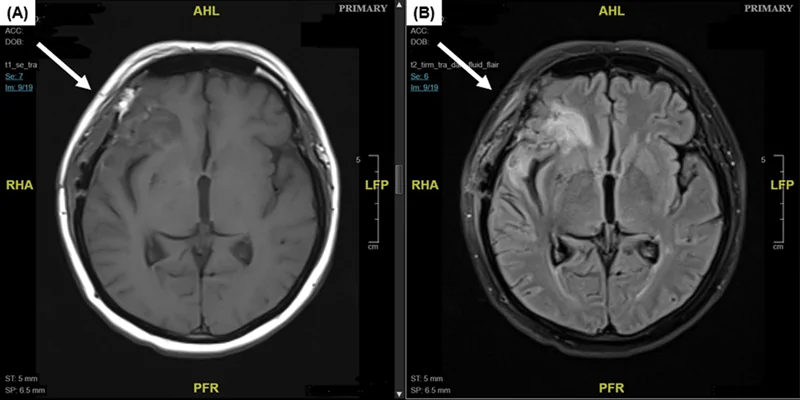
Magnetic resonance imaging (MRI) of the brain demonstrating an extra-axial mass arising from the right sphenoid wing. (
